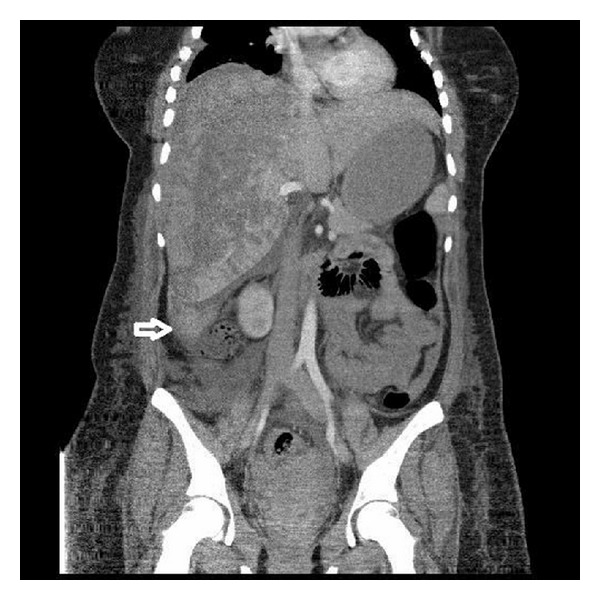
Computed abdominal tomography (CT) scan showed SLH.
